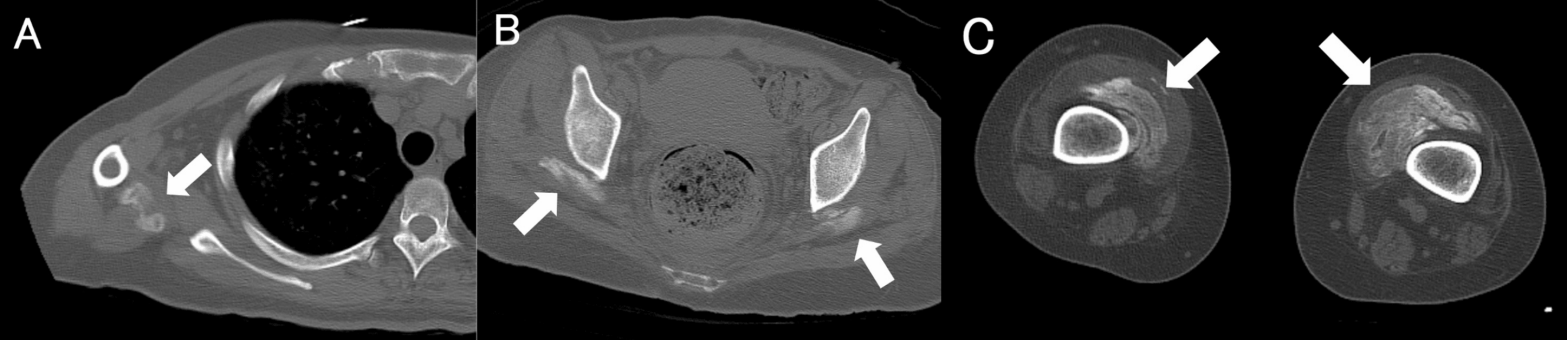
Whole-body computed tomography of immature heterotopic ossification (HO) in the right triceps brachii (A), bilateral gluteus medius (B), and vastus medialis (C).
